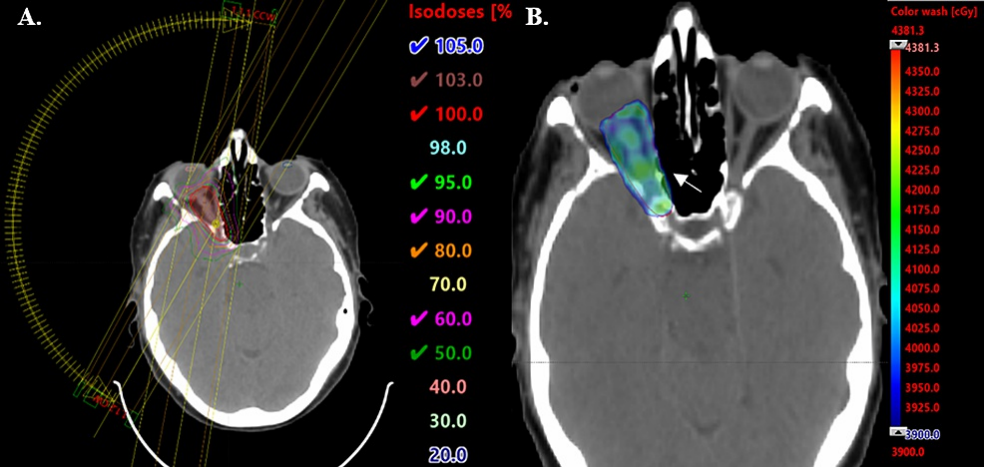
A) Axial view of treatment planning CT scan demonstrating partial arcs and the irradiated region. Isodose lines ranging from 50% to 105%. Field arrangement allows for significant reduction of radiation dose to the right orbit, pituitary gland, optic chiasm (not visualized), and contralateral optic nerve and orbit. B) Color wash showing doses above 3900 cGy. Light blue represents the prescription dose of 4000 cGy given in 16 fractions.
